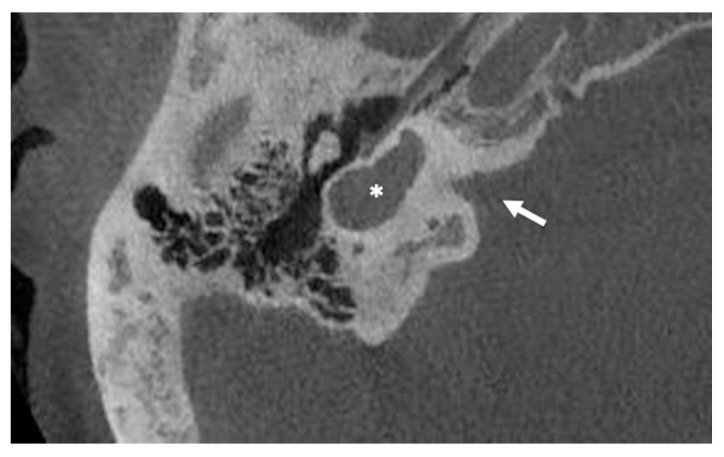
Cone beam CT imaging of a common cavity deformity (white asterisk). Note that the axis of the internal auditory canal (white arrow) seems to divide the cochleovestibular structure into anterior and posterior parts. This characteristic makes the common cavity different from the cochlea aplasia with dilated vestibule.
